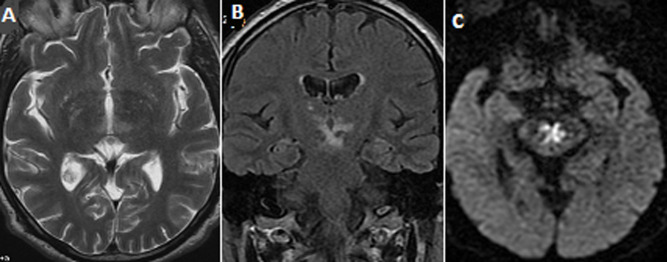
IRM cérébrale en coupe axiale T2 (A) et coronale FLAIR (B) et Diffusion (C) montrant un hypersignal mésencephalique périacqueducal et autour du 3^e^ ventricule avec restriction de la diffusion
